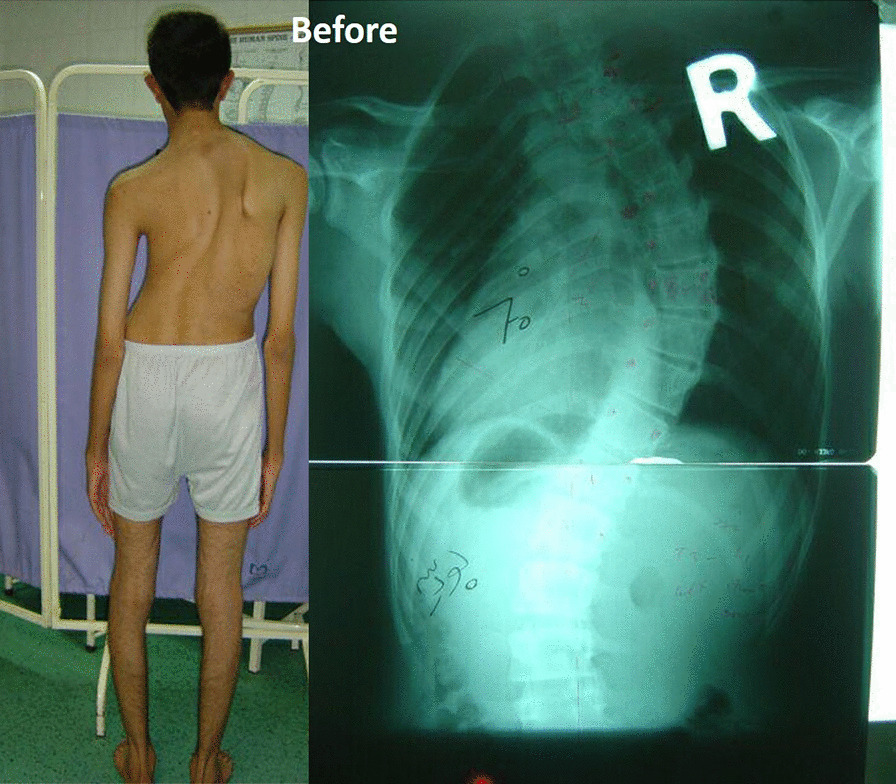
Initial radiograph. Patient with Marfan syndrome and with right thoracic scoliosis of 70°
